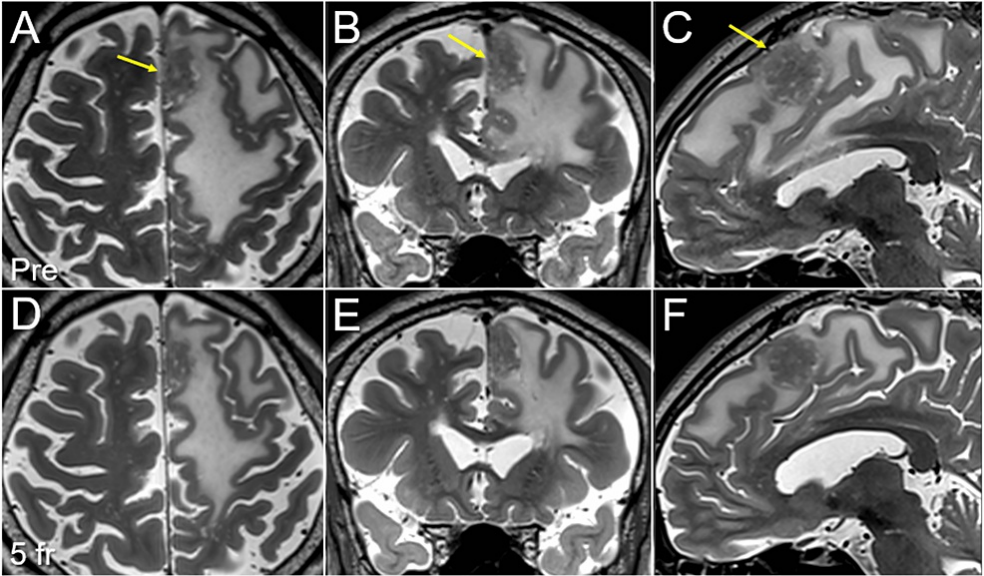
T2-weighted images before and at the completion of five-fraction stereotactic radiosurgery The images show axial images (A, D); coronal images (B, E); sagittal images (C, F); three days before initiation of SRS (A-C); and at the completion of five-fr SRS (seven days after initiation of SRS) (D-F). (A-C) The para-falcine mass lesion (arrows in A-C) is seen. (D-F) At the completion of SRS, shrinkage of the mass lesion along with mitigation of the perilesional edema and mass effect was observed. SRS: stereotactic radiosurgery; fr: fraction
